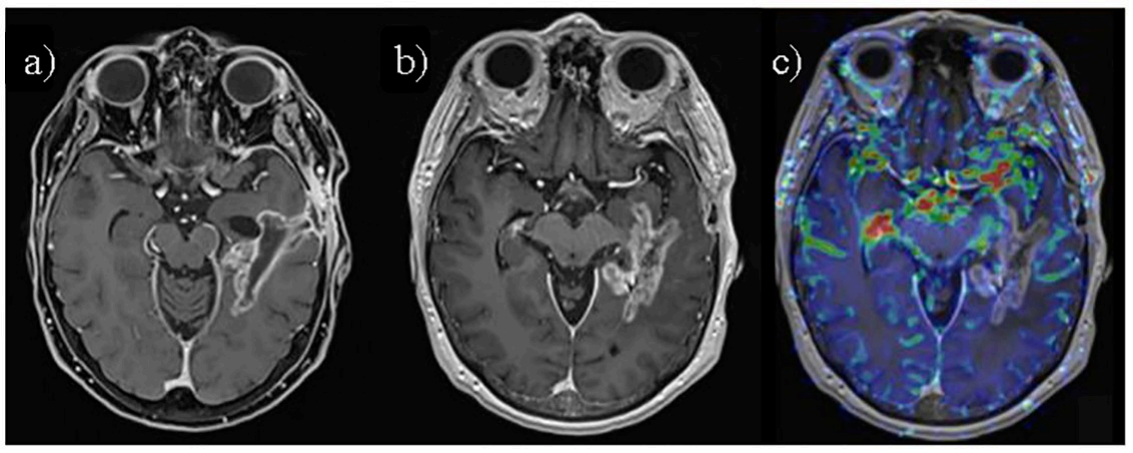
Symptomatic grade 3 radiation necrosis. a) Axial fat-saturated contrast-enhanced T1-weighted image (CE T1-WI) MRI 4 months after HFRT/TMZ, showing mostly linear enhancement surrounding the surgical cavity in left temporal lobe. b) Axial CE T1-WI of the same patient 2 months later (6 months after HFRT/TMZ), shows increased enhancement with a "soap-bubble" pattern, suggestive of radiation necrosis/pseudoprogression. c) Axial perfusion-weighted cerebral brain volume (CBV) map fused over CE T1-WI shows decreased perfusion values in the enhancing area, further supporting radiation necrosis.
